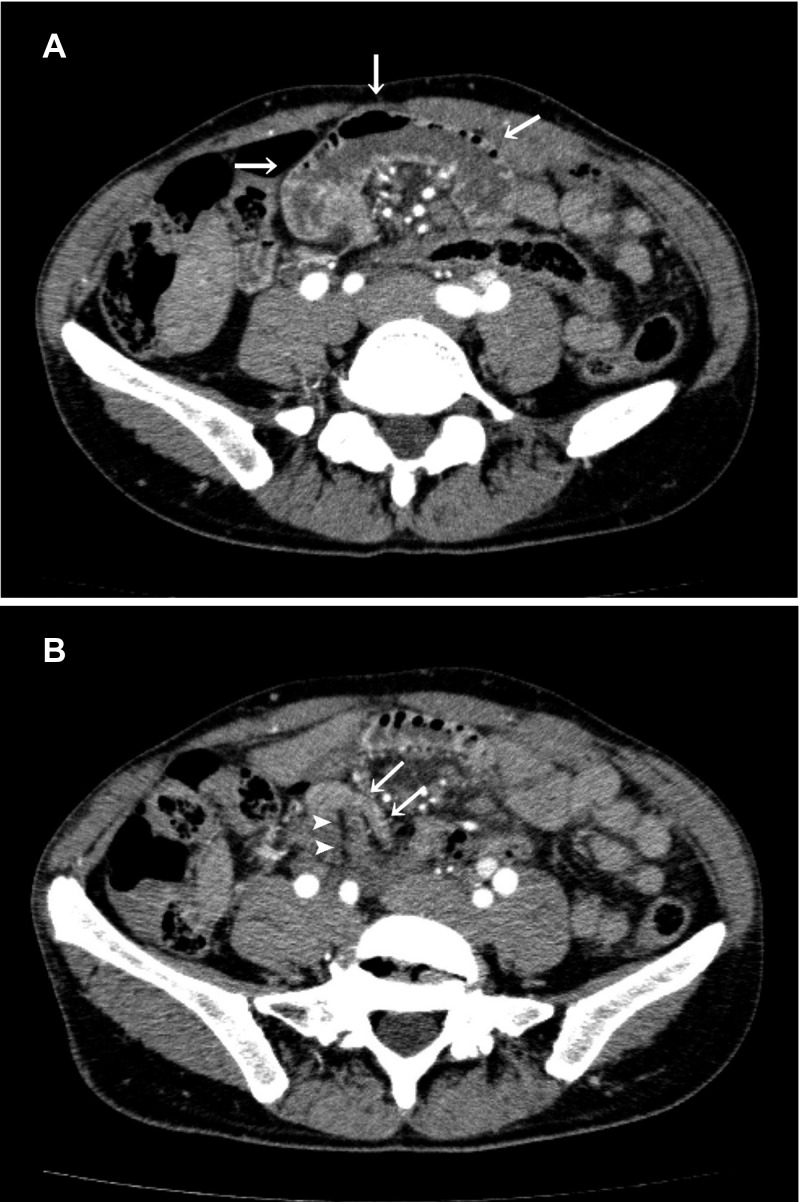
(A) Contrast-enhanced computed tomography scan of the abdomen showing a dilated upper intestine (arrows). (B) A band near the stenosis (arrowheads) and a collapsed intestine (arrows).
